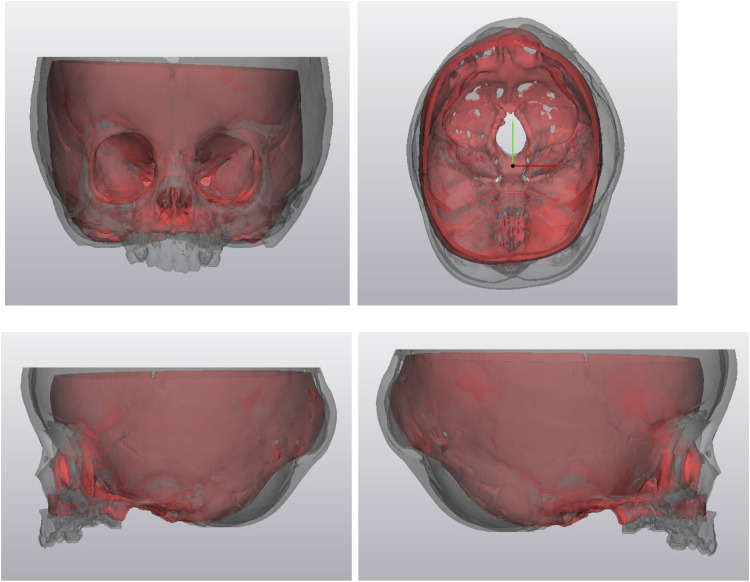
CT Overlay. Clockwise from top Left: Anterior, Superior, Right Lateral, and Left Lateral Views. Red Model is Pre-Operative and Grey is Post-Spring Removal. The two Scans Were Aligned Based on the Sella turcica.
